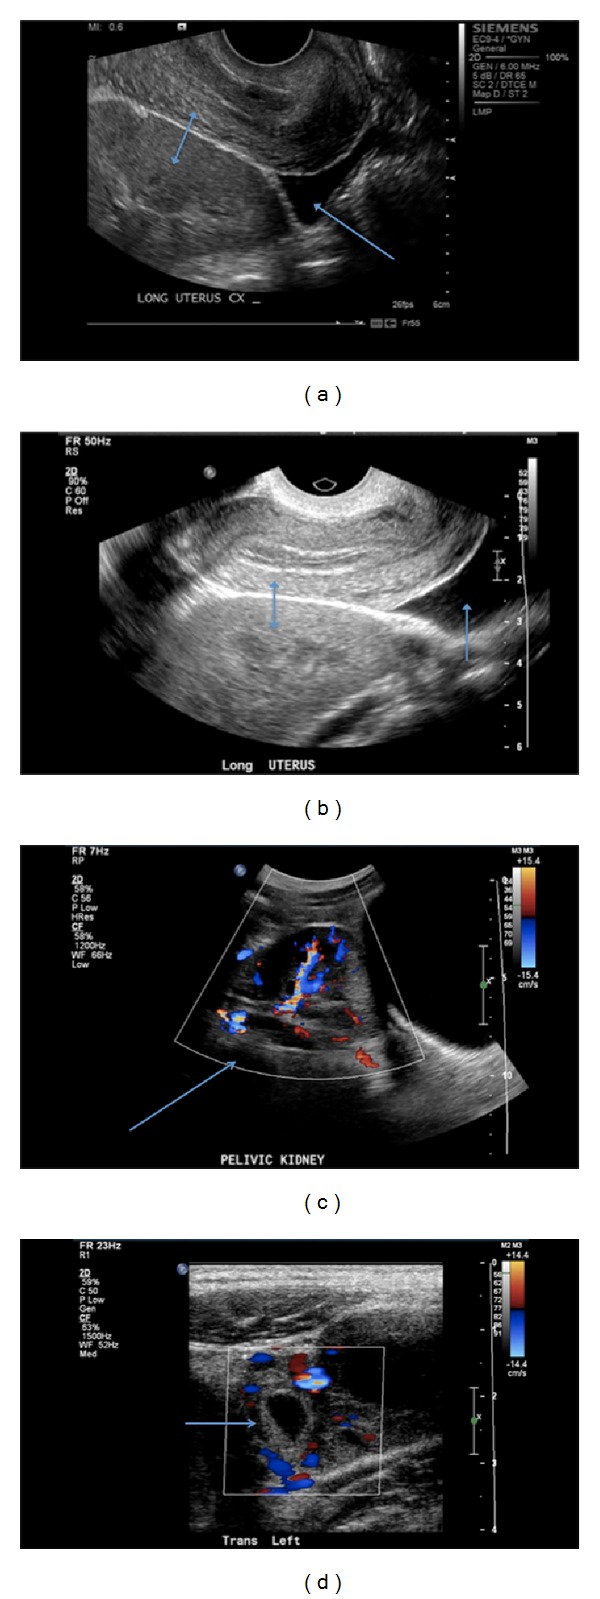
(a) Longitudinal view: an empty uterus superiorly and pelvic kidney inferiorly (double arrow), free fluid in the cul-de-sac (single arrow). (b) Longitudinal view: an empty uterus superiorly and pelvic kidney inferiorly (double arrow), free fluid in the cul-de-sac (single arrow). (c) Longitudinal view: ectopically located pelvic kidney with color Doppler (single arrow). (d) Transverse view: hypoechoic cystic structure with vascular flow in the left adnexa depicting a tubal pregnancy (single arrow).
